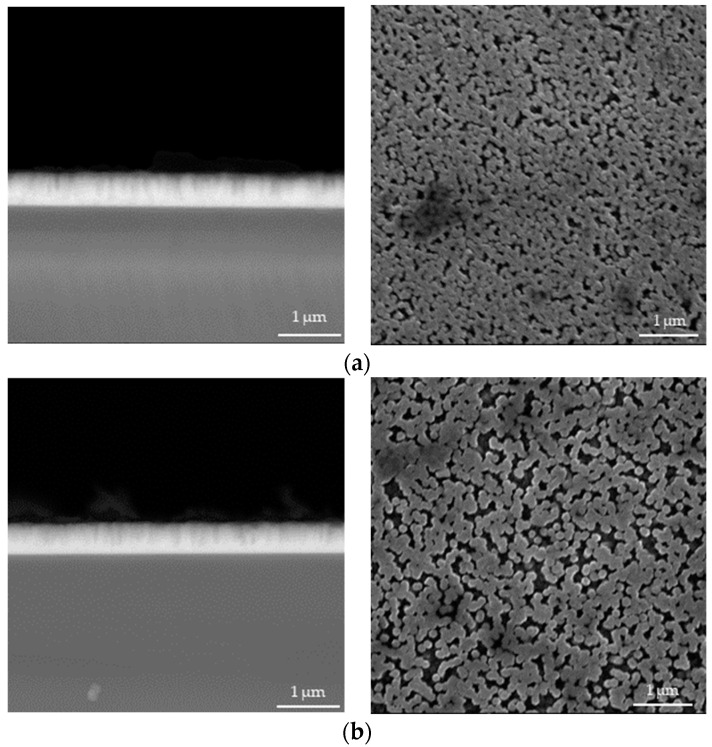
SEM images of nanorods obtained with 2 min of ultrasound and dried for 1.5 h (a) and 2.0 h (b) (left: cross-section image; right: surface image).
